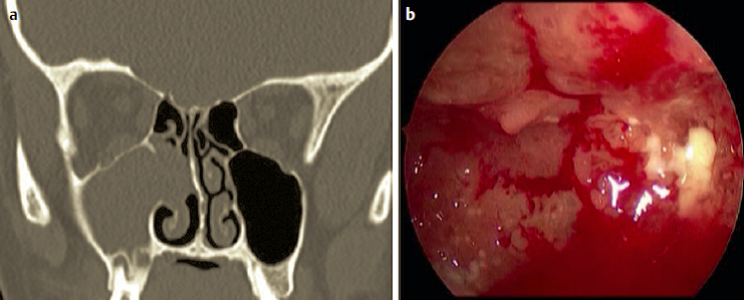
Chronic maxillary sinusitis of the right side. The causative preapical abscess was not identified neither by the dentist nor by the radiologist (CT scan). The papillomatous thickened mucosa can and should be preserved (intraoperative endoscopy, 45° optics).
